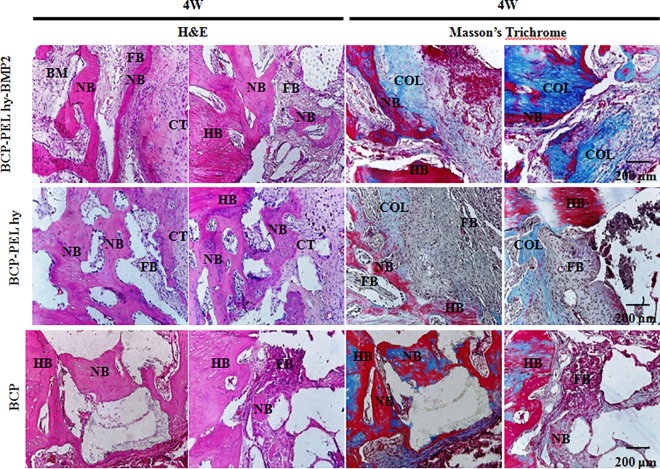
Cellular activity at scaffold-host bone interphase at 4 W time period. Here HB, NB, BM, CT, FB and COL stands for host bone, new bone, bone marrow, cartilage template and collagen.
